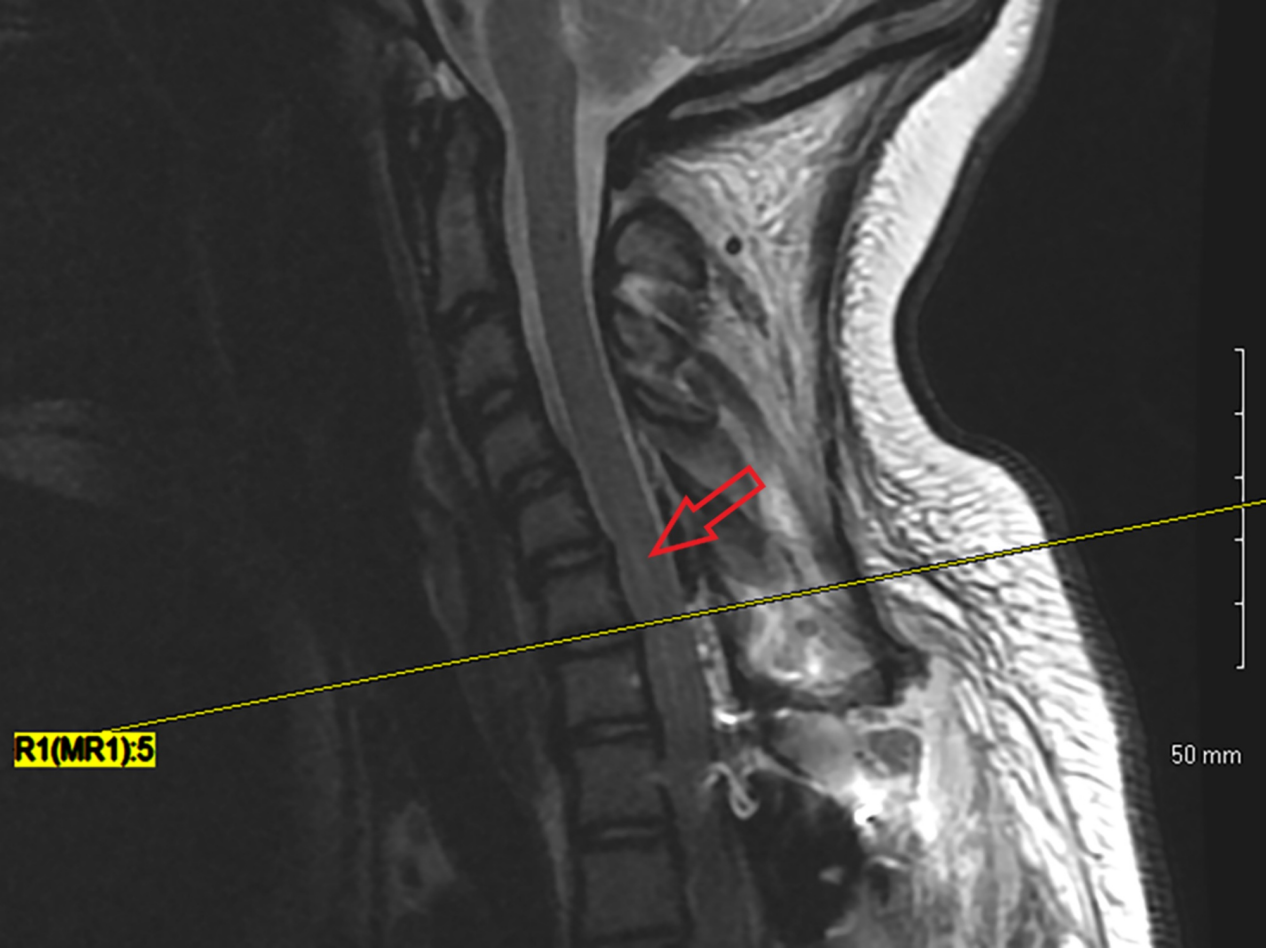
Postoperative sagittal MRI demonstrating acute myelomalacia changes of the spinal cord
